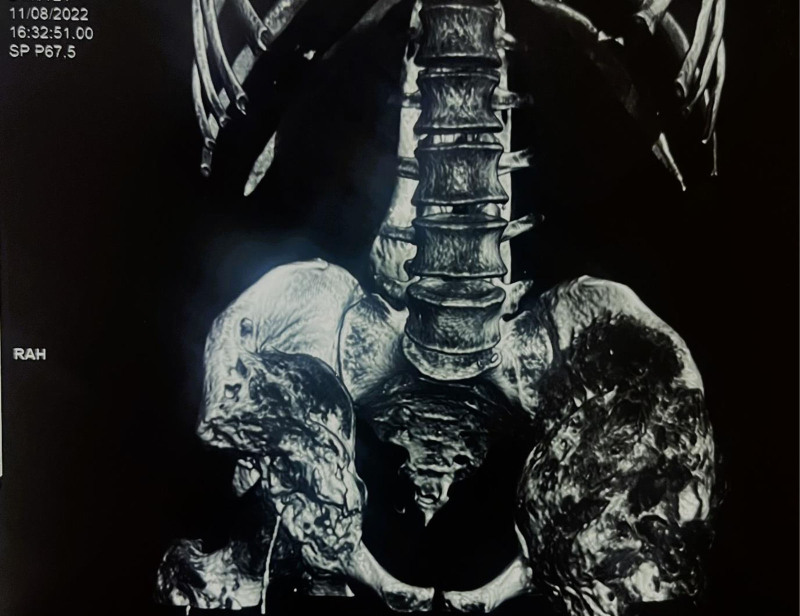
CT 3-dimensional reconstruction of spinal muscles and hips, a year after surgical treatment, showing reoccurrence of the tumor. CT = computed tomography, RAH = Rehaussement annulaire en Halo.
